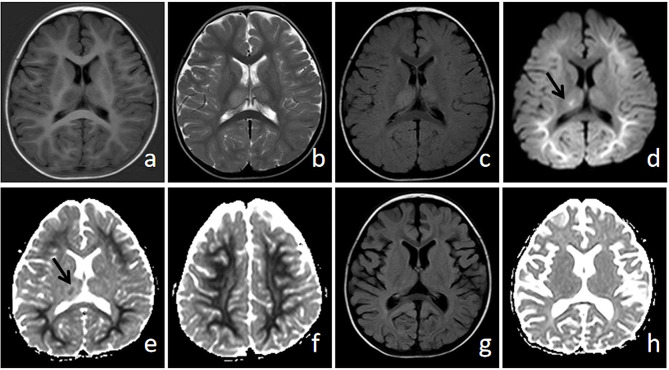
MRI results of Patient 5 One year and ten months old gairl, was admitted for “six days of cough, intermittent fever and convulsions for five days”. On the 8th day of the disease course, the brain MRI showed flaky long T1 (A) and long T2 (B) abnormal signals in the bilateral thalamus, T2 FLAIR (C) had a high signal, and small patchy diffusion restriction (D, E arrows) could be seen in the right thalamus lesion. Diffusion restriction (D ∼ F) could be observed in the bilateral cerebral hemisphere and subcortex, showing “bright tree signs”, indicating cytotoxicoedema. Re-examination of the brain by MRI on the 19th day of the disease showed brain atrophy-like changes in T2 FLAIR images (G), the abnormal signals in the bilateral thalamus had basically disappeared, and the apparent diffusion coefficient (ADC) (H) indicated that there was no restriction of diffusion in the brain
